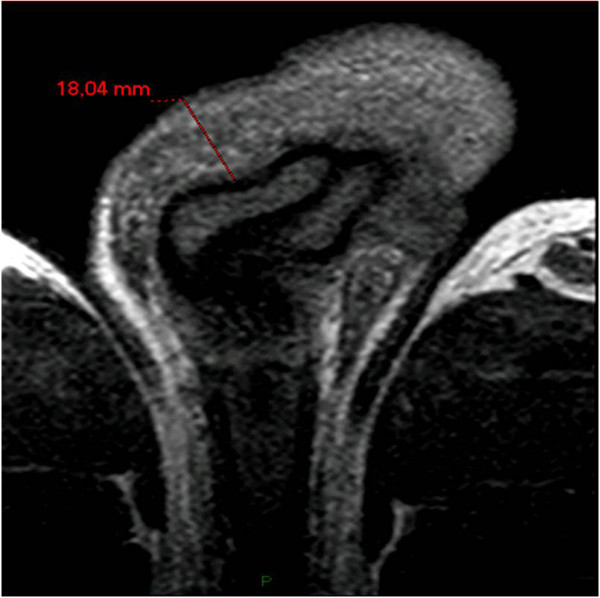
Axial T1-weighted TSA MR imaging shows iso-hypointense 18 mm tissue (red line) surrounding the corpora cavernosa of the penis.
